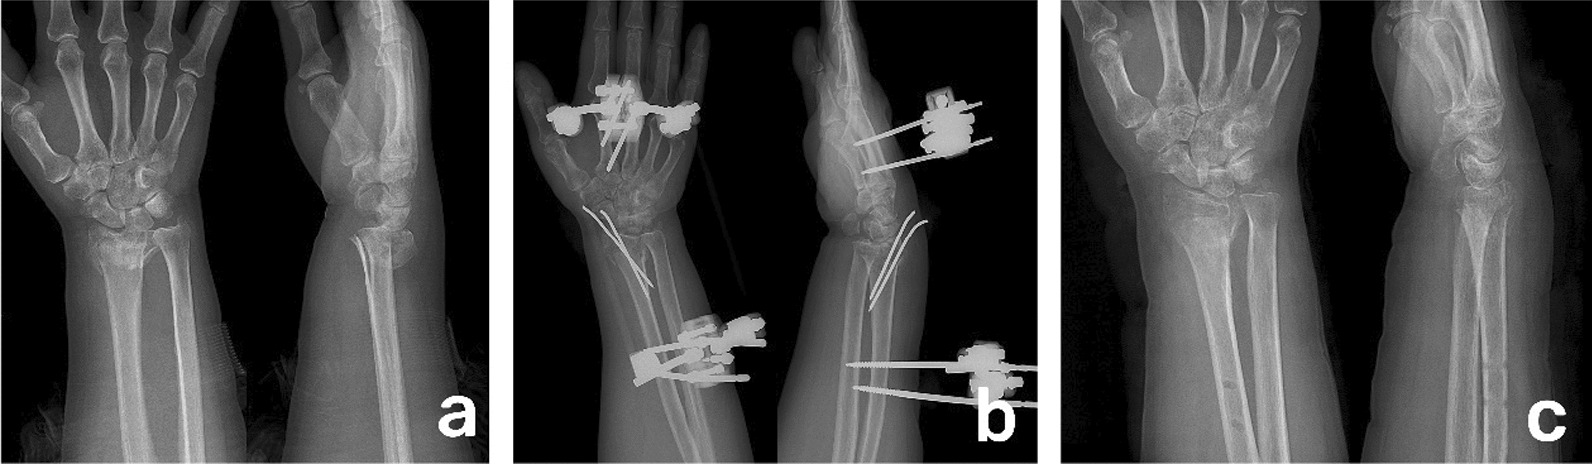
a Preoperative anteroposterior and lateral X-ray films of a patient with C3 type distal radius fracture. b Anteroposterior and lateral X-ray films on the first day after operation, showing adequate reduction and external fixation combined with Kirschner wire fixation of DRF. c. Anteroposterior and lateral X-ray films taken after 6 weeks, showing removal of external fixator and Kirschner wire
